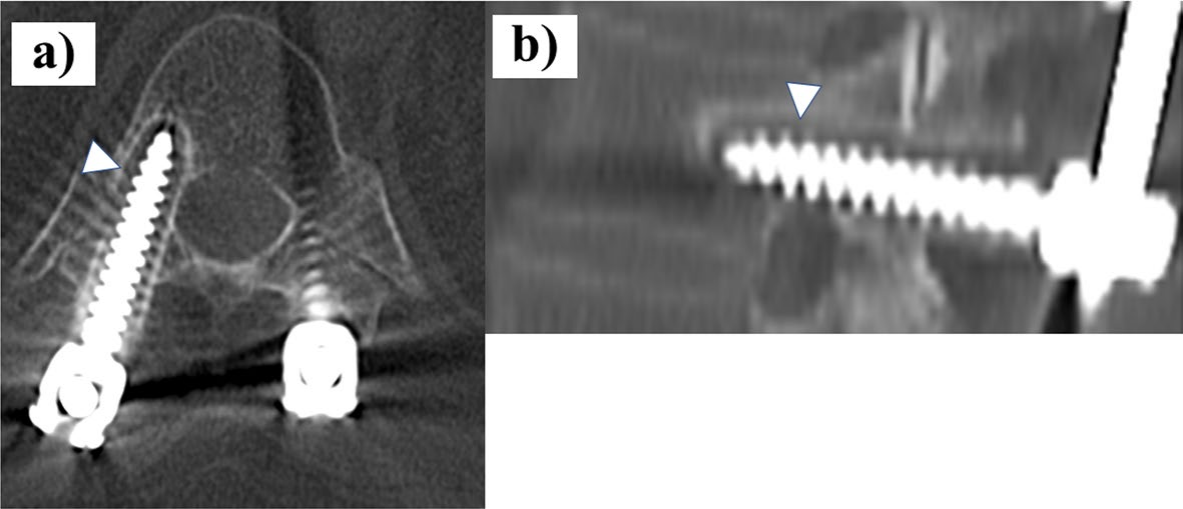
Axial (a) and sagittal (b) CT scan of a patient subjected to thoracolumbar posterior fixation showing a radiolucent area and the halo sign, indicative of screw loosening, indicated by the white arrowheads
